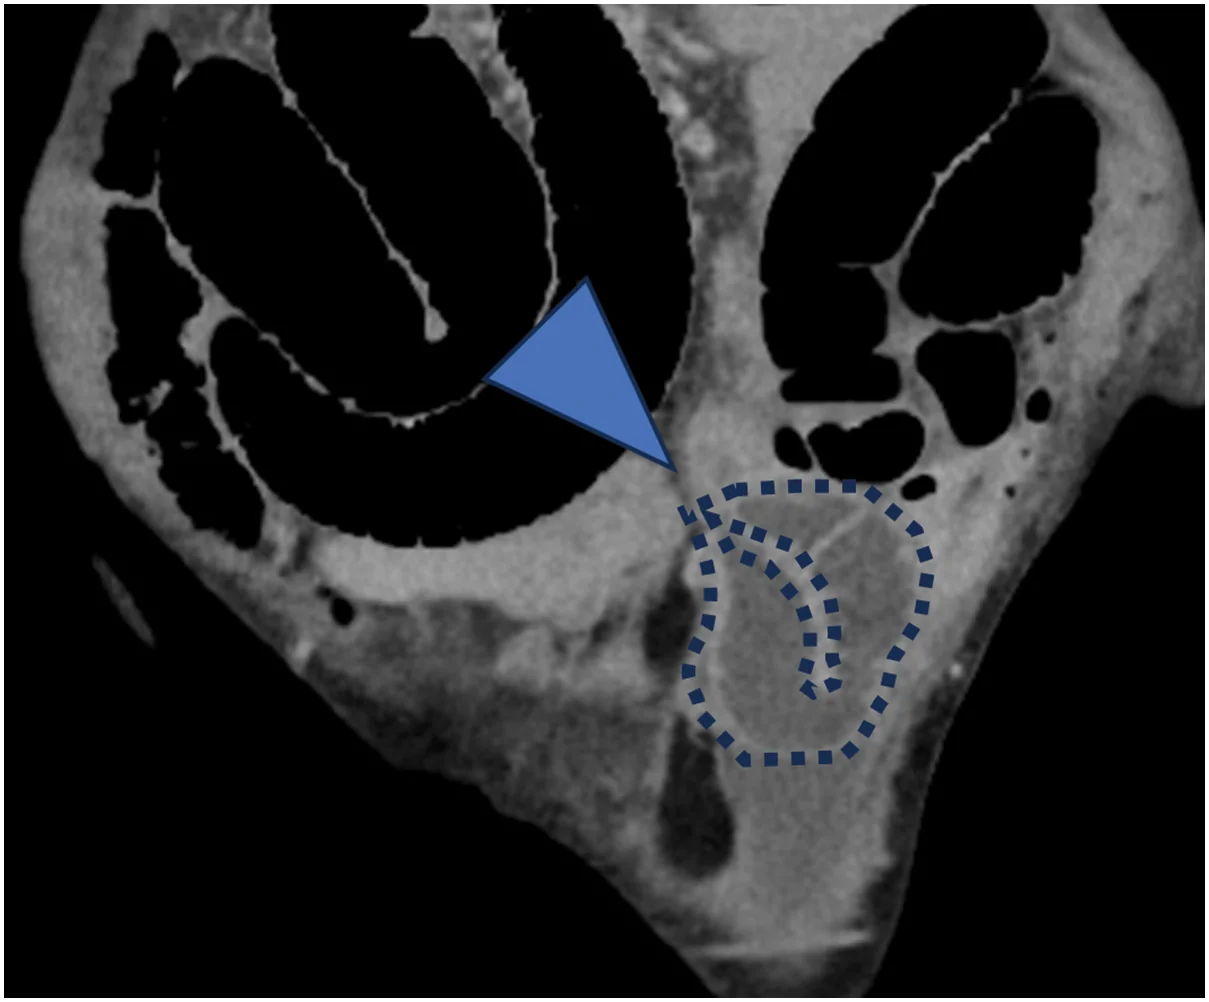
Retrospective review of post-reduction contrast-enhanced CT imaging. Retrospective review of the post-reduction CT demonstrating subtle findings consistent with a closed-loop obstruction. Dotted lines outline the closed-loop segment, while the arrowhead indicates the constricting site (transition point). Bowel wall enhancement remained preserved, and no definitive signs of ischemia were identified.
